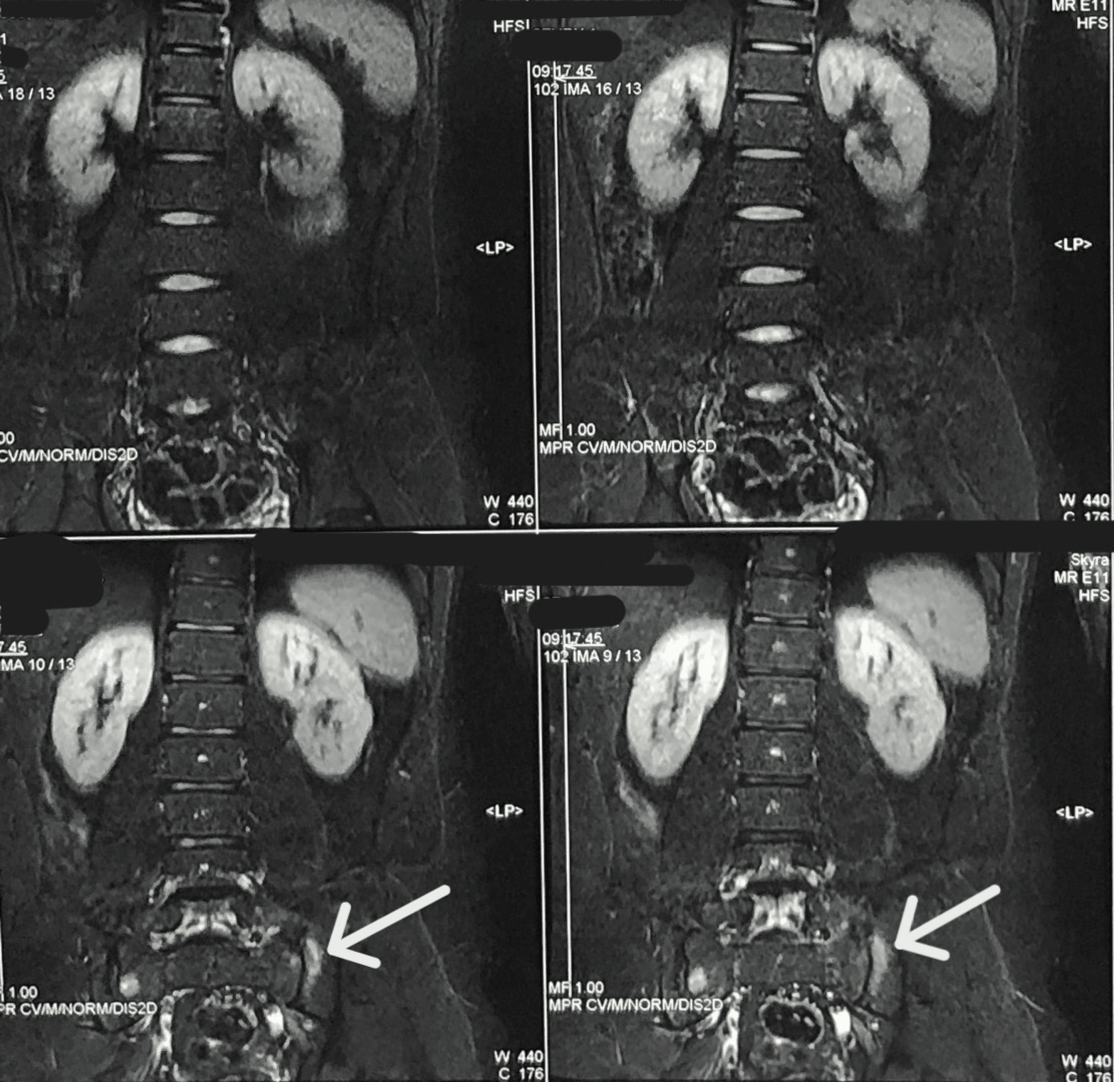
MRI showing sacroiliac joint space reduction with articular surface irregularity and bone marrow edema.
